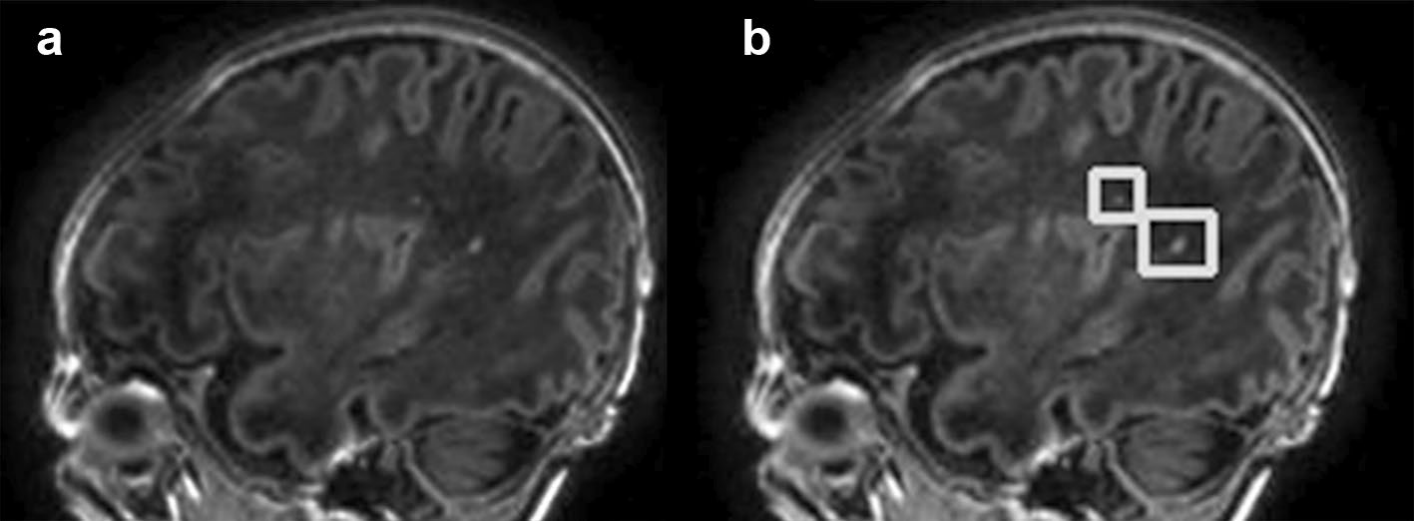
Automatic detection of punctate white matter lesions (PWMLs) in an infant: two PWMLs in a sagittal T1-weighted image (a) are correctly detected (b, squares).
